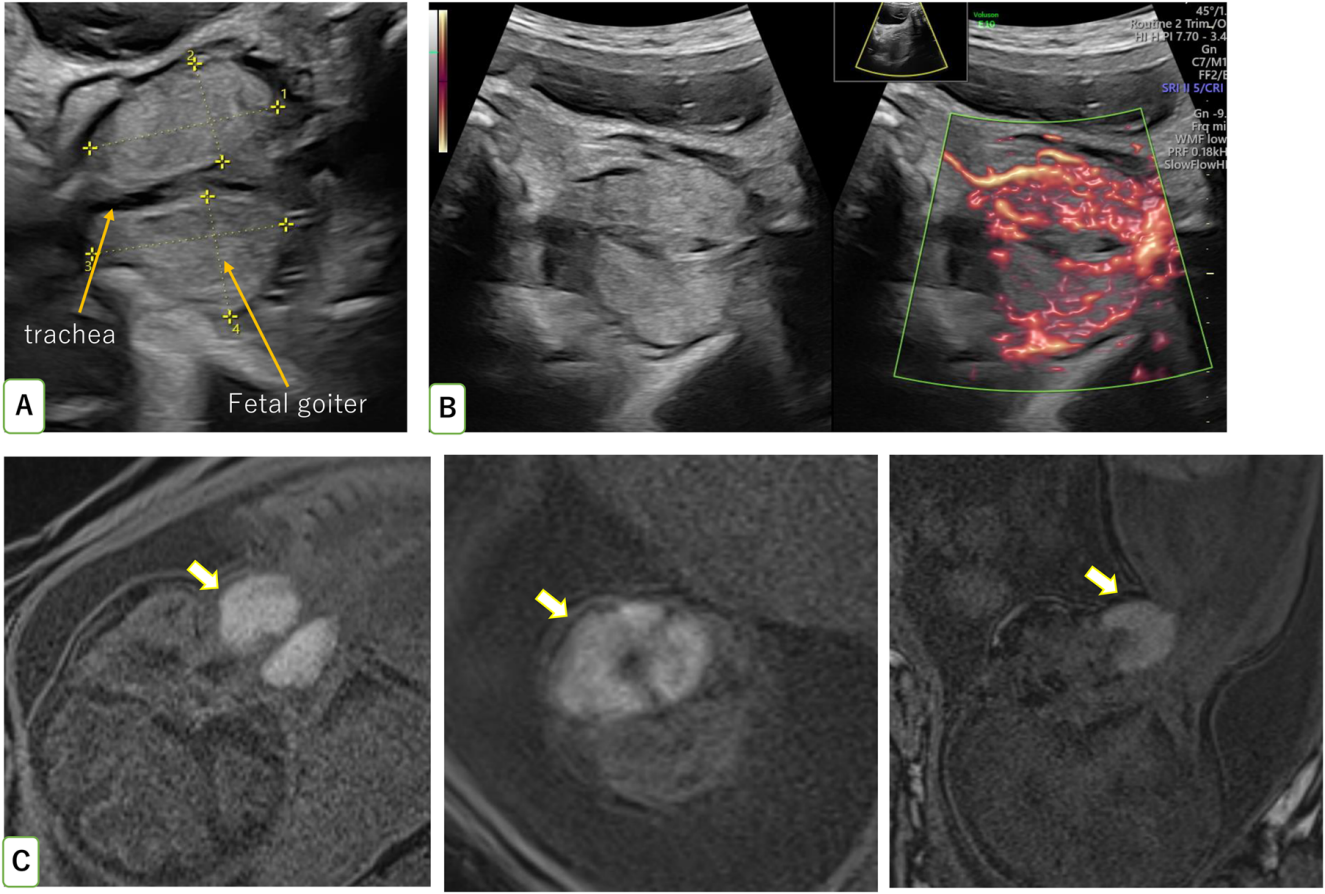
Ultrasonographic examination and magnetic resonance imaging (MRI) of the fetal goiter at 33 weeks’ gestation. (A) Size of the right thyroid lobe was 2.9 × 1.6 cm and that of the left thyroid lobe was 2.9 × 1.9 cm in the anterior aspect of the fetal neck. (B) On color Doppler examination, the vascularization of the goiter was concentrated in the periphery. (C) On fetal magnetic resonance imaging (MRI) T1-weighted image shows a massive fetal goiter.
